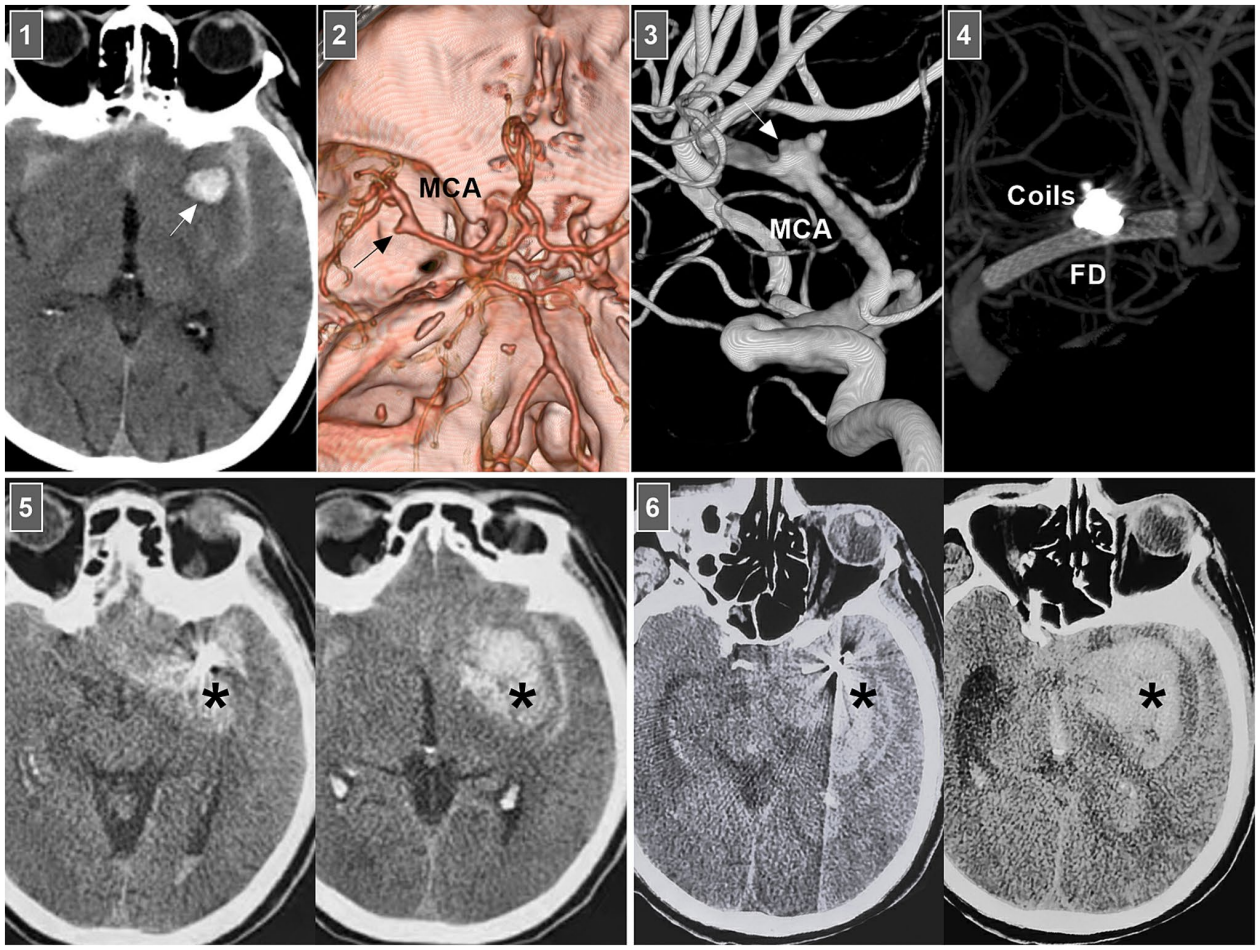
Rebleeding after EVT of an MCA dissection. Panel 1: CT showing subarachnoid hemorrhage and parenchymal hematoma (arrow). Panel 2: CT angiography showing a left MCA trunk aneurysm (arrow). Panel 3: DSA showing that the aneurysm (arrow) was an MCA trunk dissection involving the origin of lenticulostriate artery. Panel 4: Vaso-reconstructive image showing that MCA dissection was coiled by FD assistance. Panel 5: Postoperative 1-h CT images showing increased hemorrhage (asterisks), indicating the MCA dissection reruptured. Panel 6: Postoperative 3-h CT images showing the bleeding (asterisks) continued to increase, the patient fell into coma. CT, computed tomography; DSA, digital subtraction angiography; EVT, endovascular treatment; FD, flow diverter; MCA, middle cerebral artery.
